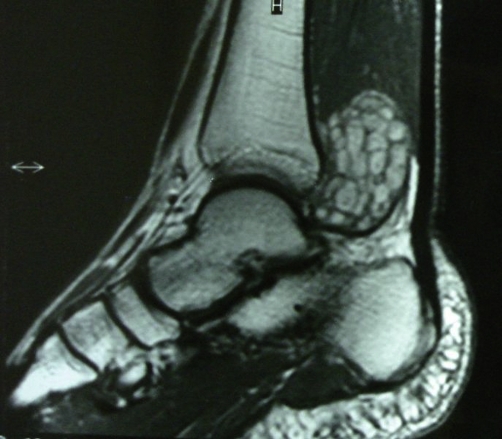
Preoperative T1-weighted MR image demonstrates a multinodular tumor of the posterior tibial nerve.
